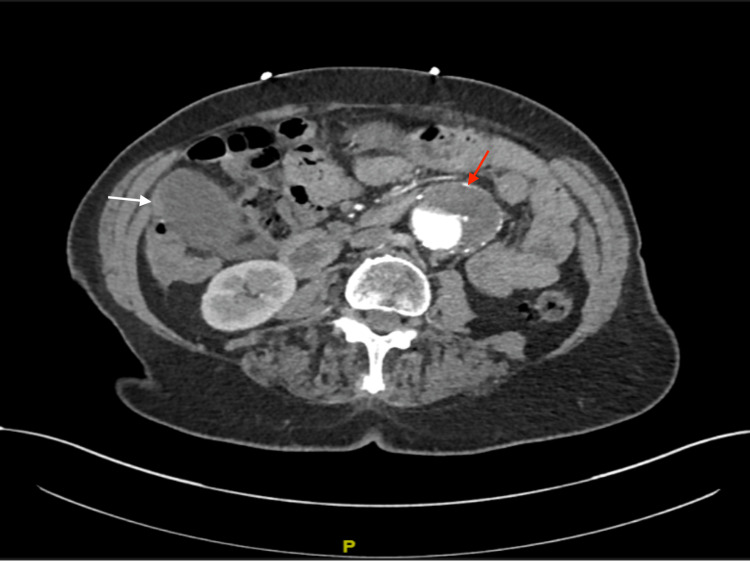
CTA abdomen and pelvis Computer tomography angiography (CTA) abdomen and pelvis showing concomitant 5.5 cm infrarenal abdominal aortic aneurysm and distended gallbladder with wall thickening concerning cholecystitis.
